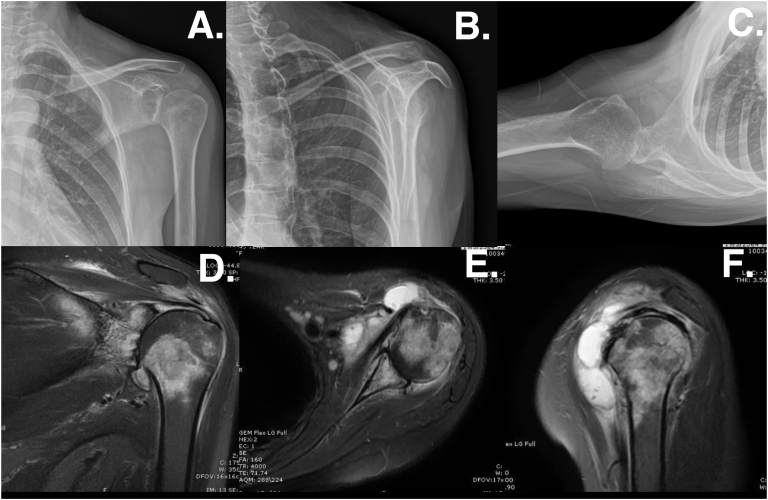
The radiographic imaging of the left shoulder showed that the narrowing and attrition between glenoid and humeral head a.) Anteroposterior view, b.) Transcapular Y view and c.) Transaxillary view. The MRI T2FS of the left shoulder showed d.) Coronal view T2FS, e.) Axial view T2FS and f.) Sagittal view T2FS.
